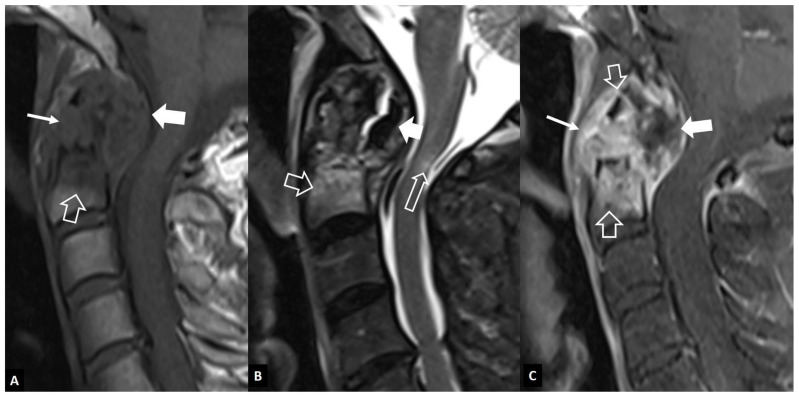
A 64-year-old male patient with long-lasting rheumatoid arthritis of the hands and wrists and progressive onset of pain, increased reflexes and numbness in the arms, and neck pain, 5 months prior to imaging. Sagittal MR images. (A) T1-w image showing bone marrow edema in the C2 vertebral body (open arrow), cortical disruption and soft-tissue changes in the anterior odontoid process (thin arrow), and an intermediate signal intensity mass posterior to C2 (thick arrow) compressing the spinal cord. (B) T2-w image showing to better advantage the bone marrow edema (open arrow), the low signal intensity mass posterior to C2 (arrow), suggesting pannus formation and a small area of edema within the spinal cord (long open arrow). (C) The fat-suppressed contrast-enhanced T1-w image shows the enhancement of the bone marrow edema (open arrows) and the prevertebral changes (thin arrow). The pannus tissue is only peripherally enhancing (thick arrow).
